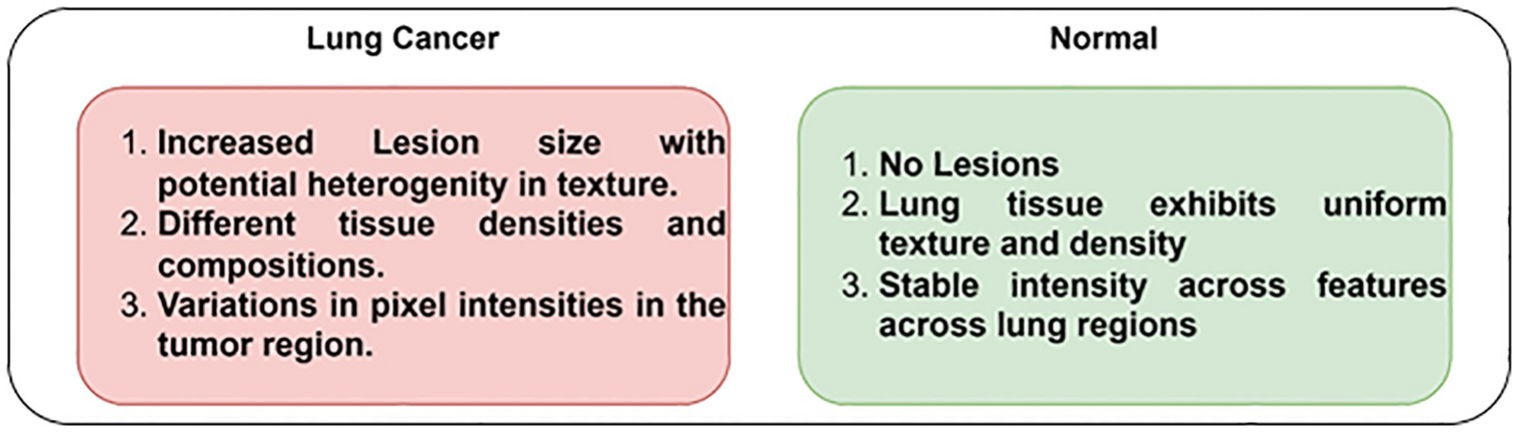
Significant features of Lung Cancer and normal PET / CT images.
